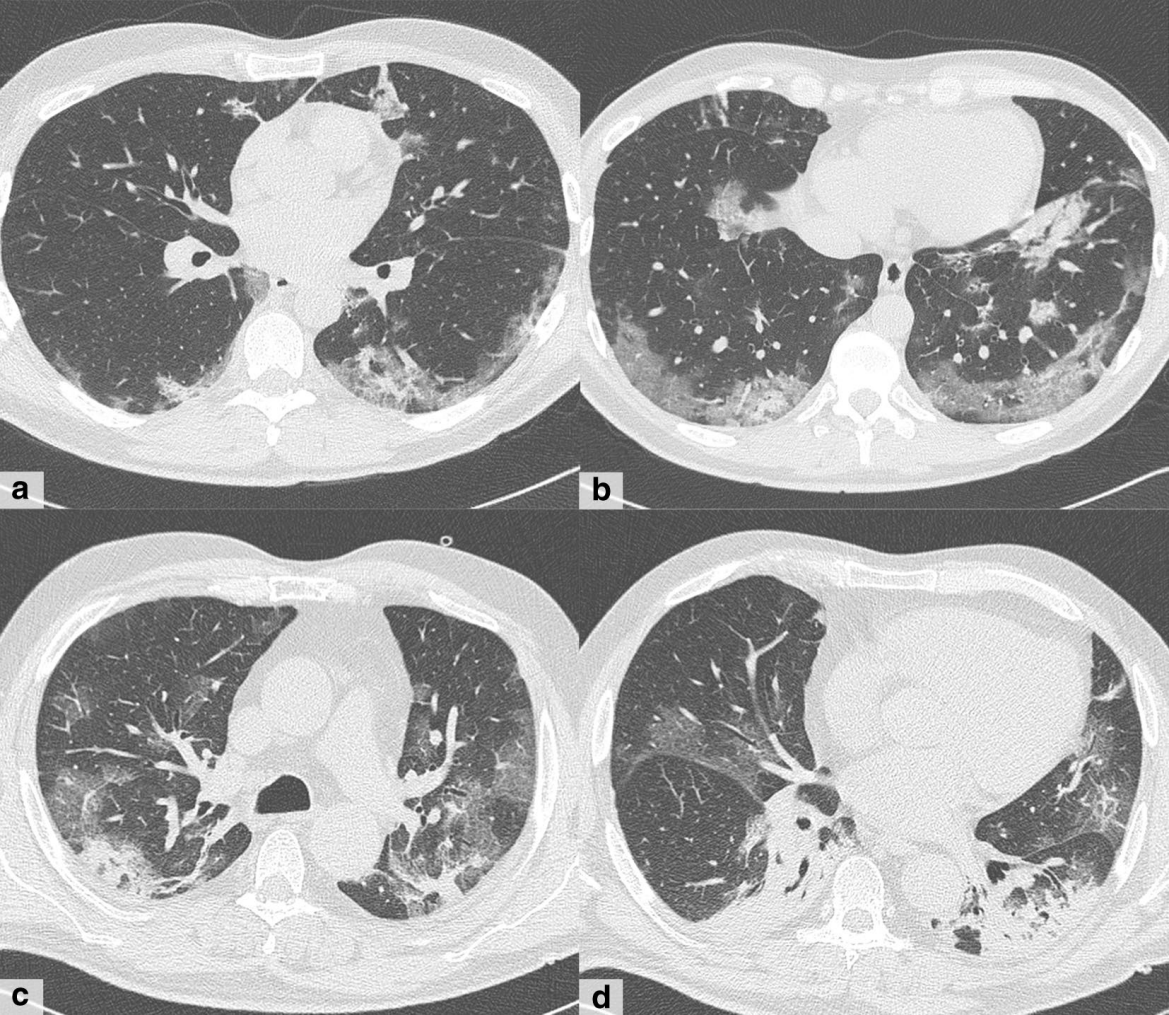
a–b CT scan in a 43-year-old man with fever and cough in the last 12 days shows the most typical and frequent features of COVID-19 pneumonia: bilateral multifocal and confluent ground-glass opacities in a peripheral subpleural distribution, associated with consolidation area in the left lower lobe. The patient was positive to RT-PCR test. c–d A 64-year-old man with cough and dyspnea for 10 days, treated at home with antibiotics without benefit and arrived to the hospital for the onset of fever in the last day. CT scan shows similar pattern and distribution of patient in figures a, b, but the first two swabs were negative. Anyhow, he was hospitalized and treated as a positive patient. The RT-PCR test turned positive only on the third sample
